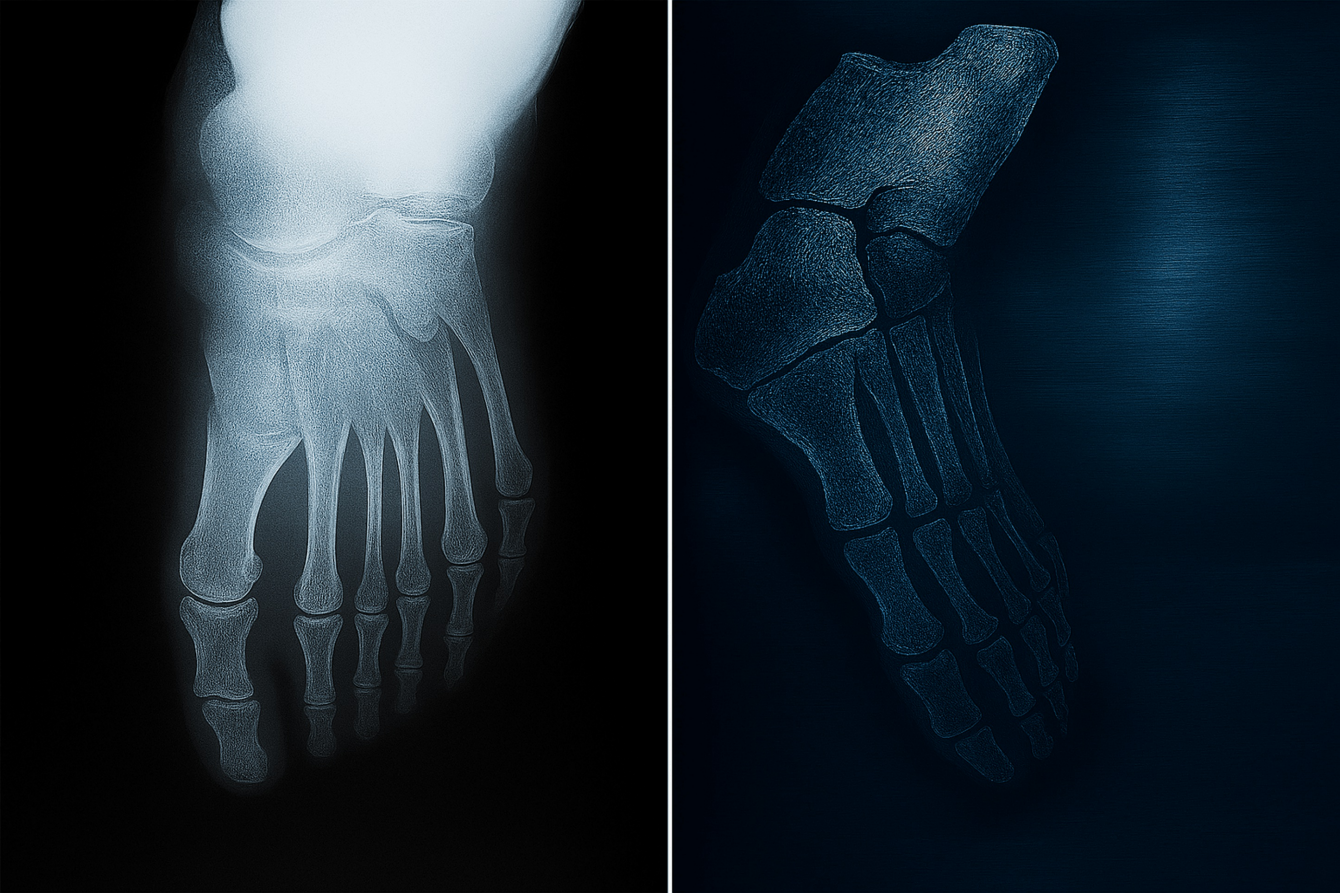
X-ray showing no abnormalities in the patient’s foot
